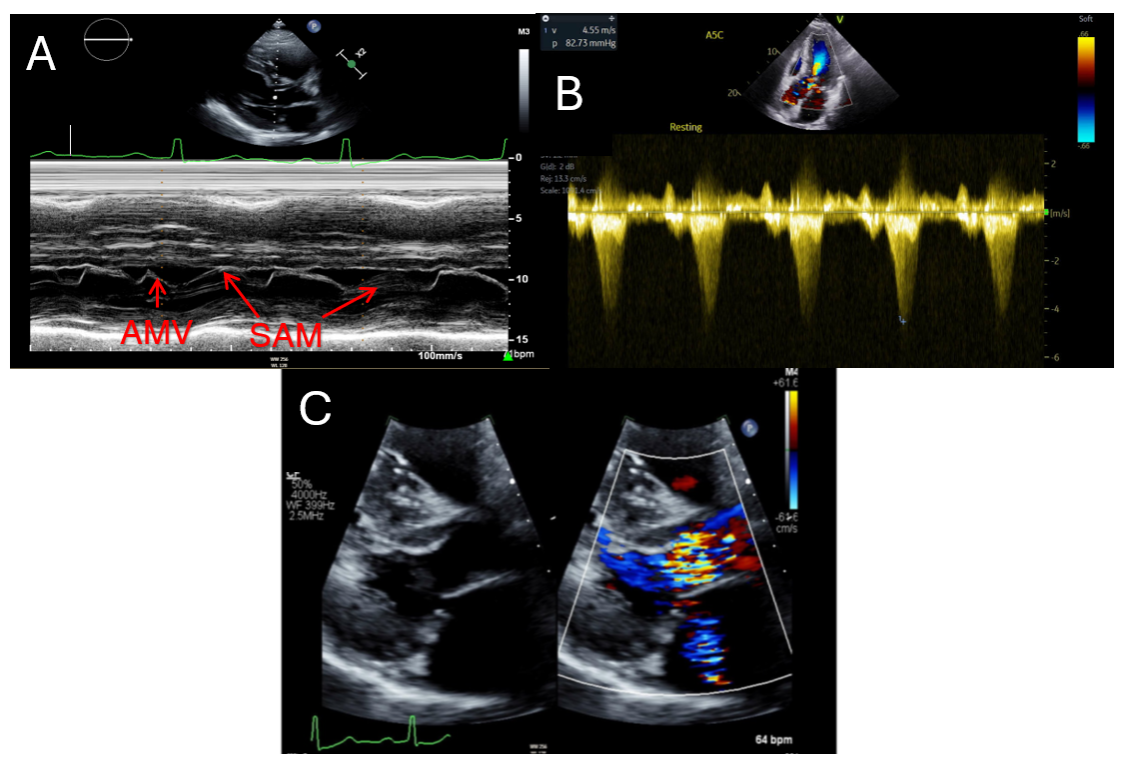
Echocardiographic findings in hypertrophic
